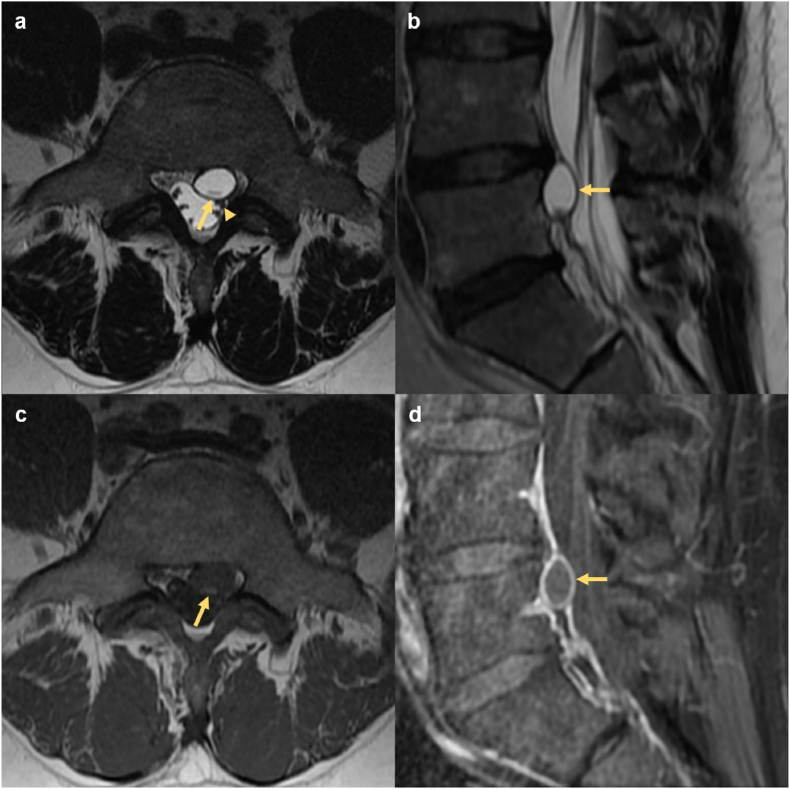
MRI in a 40-year-old male with 6 months of left-sided L5 radicular pain. (a) Axial T2W sequence demonstrates a left paracentral discal cyst (arrow) effacing the left lateral recess and displacing the descending left L5 nerve root dorsally (arrowhead). The cyst is clearly separate from the adjacent left facet joint. (b) Parasagittal T2W sequence demonstrates central T2 hyperintensity within the lesion and a T2 hypointense rim (arrow). (c) Axial T1W demonstrates that the cyst is predominantly T1 hypointense with dependent T1 isointense contents (arrow). (d) Parasagittal post-gadolinium T1W with fat suppression confirms smooth enhancement of the cyst wall (arrow).
